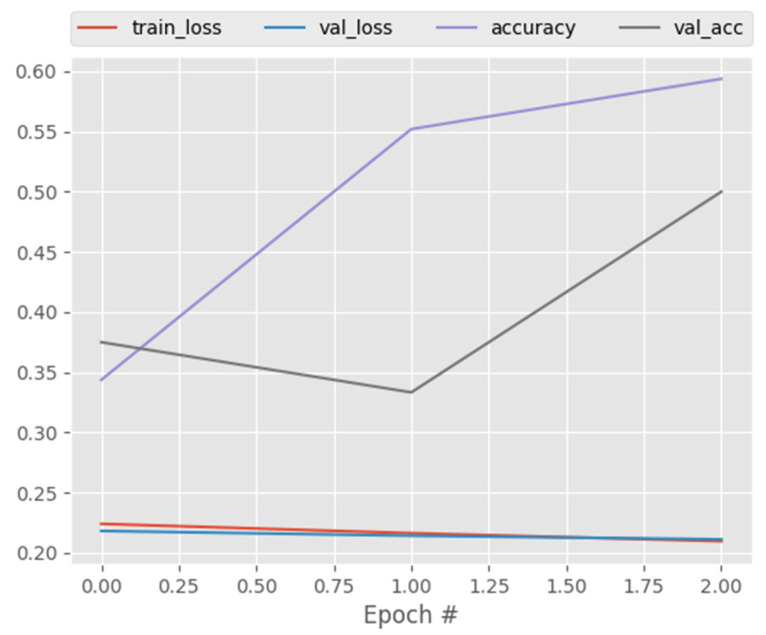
CNN training and validation performance for X-ray images.
